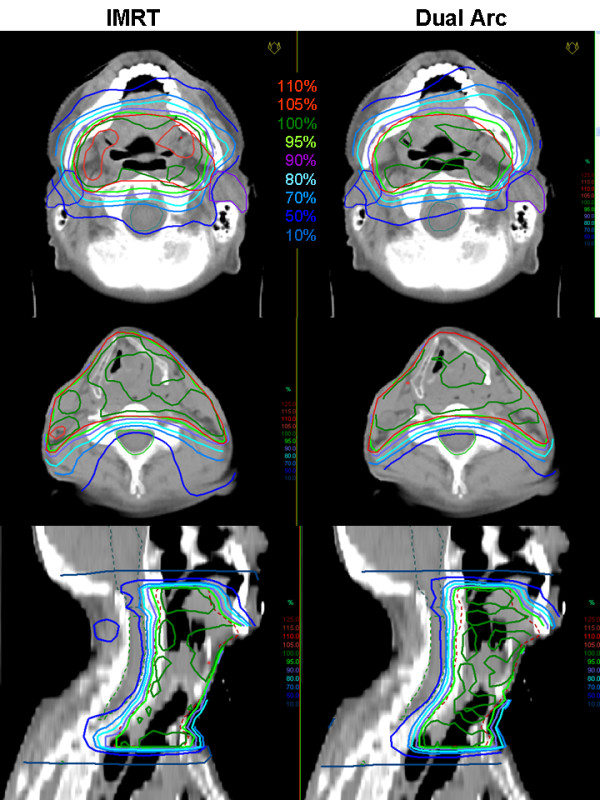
Dose distributions for hypopharynx/larynx cancer. Comparison of dose distributions achieved with 7-field IMRT (left) and Dual Arc VMAT (right) on representative transversal (top) and sagittal (bottom) CT slices. The PTV is drawn in red, the parotids in blue and purple, the spinal cord in green, and the brain stem in bright blue. Isodose lines are shown in percent of the prescription dose, i.e. 60 Gy to the average of the PTV.
